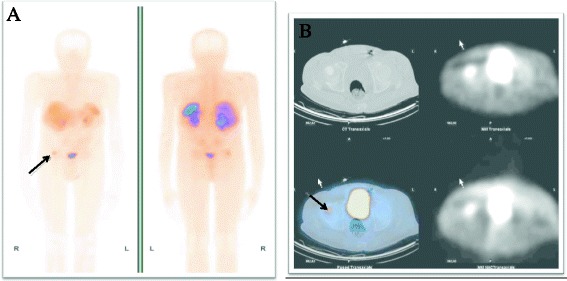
a 99mTc-Tektrotyd SPECT /CT of a pelvis. Focal, intensive radio tracer uptake in right femoral head (*long arrow*) and b corresponding sclerotic lesion on CT (*long arrow*) (year 2014)
